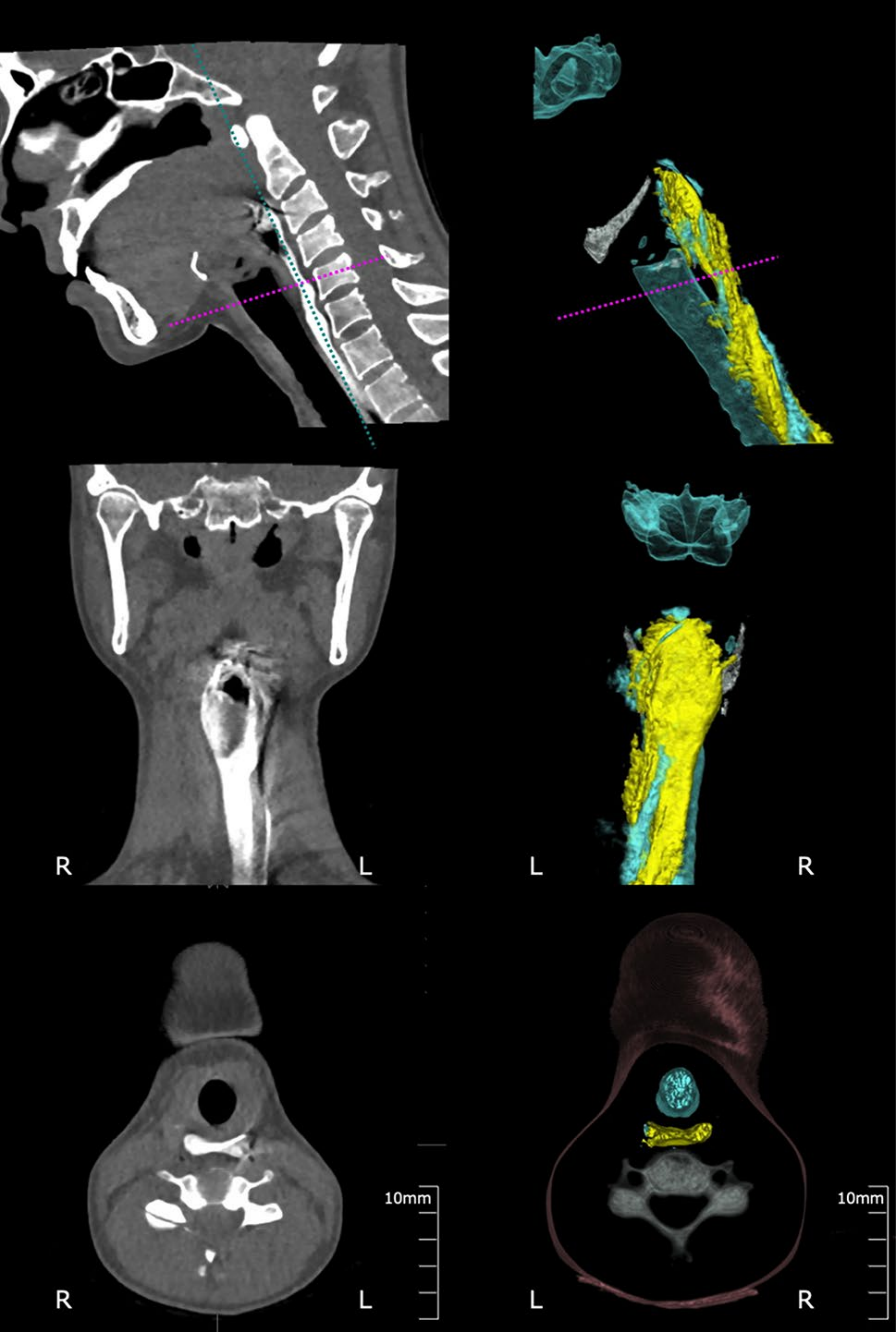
MPR images and 3D-CT images of Upper esophageal sphincter from various perspectives. Left: MPR images (upper: mid-Sagittal section, middle: coronal section, lower: axial section). Right: 3D-CT images (upper: lateral view, middle: posterior view, lower, superior view)
